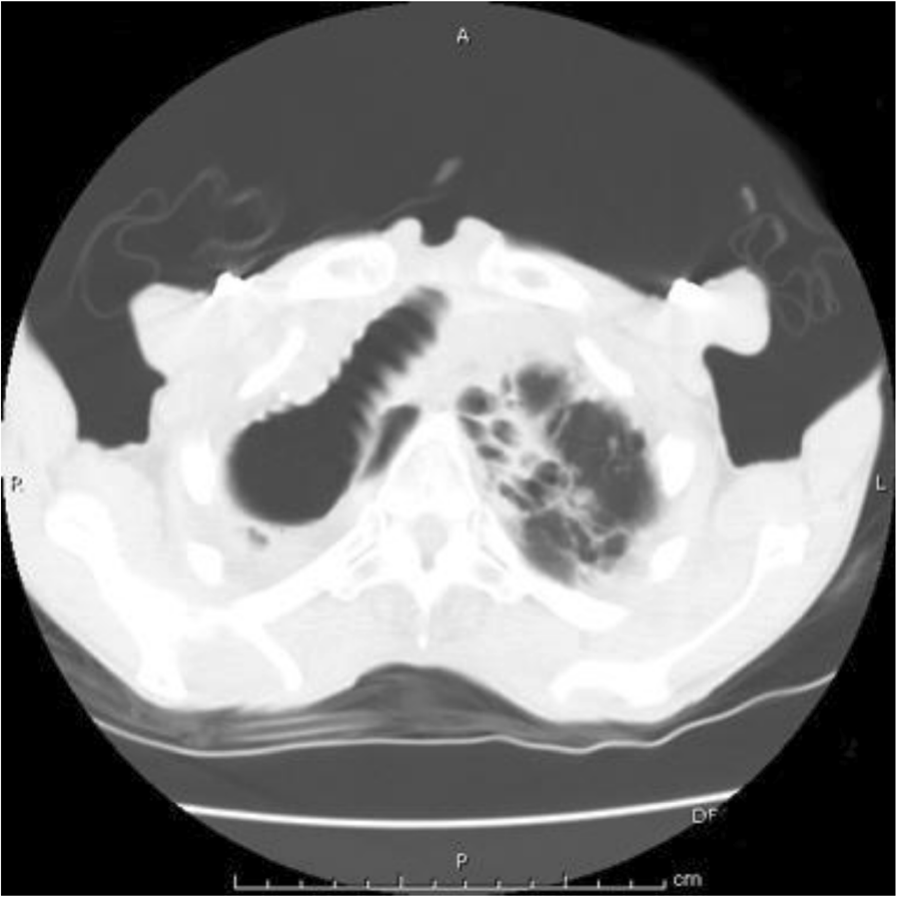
Computed tomography scan of the chest showing a dilated trachea shifted to the right hemithorax and left apical cystic bronchiectasis.
